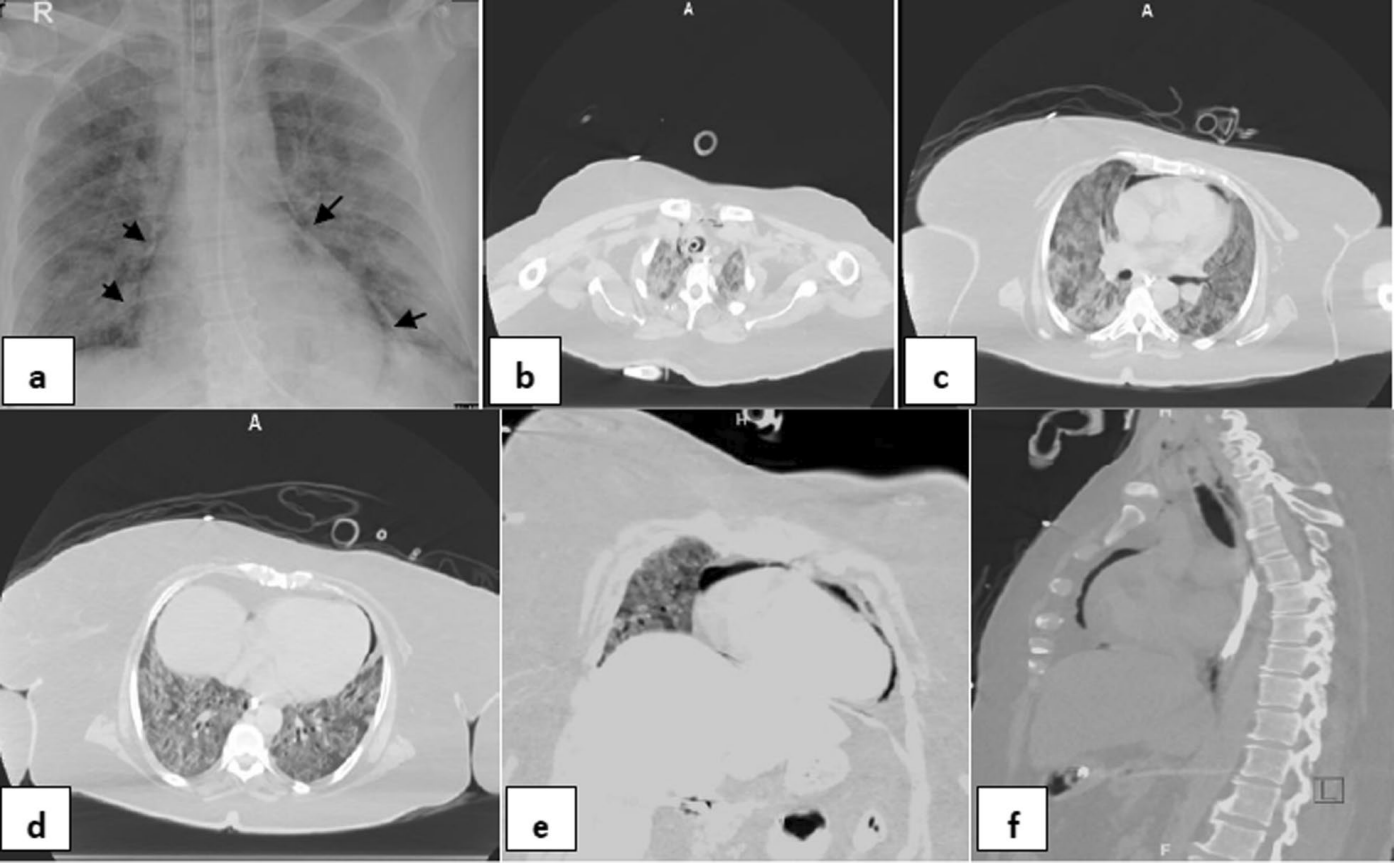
52-year-old female without significant medical history was intubated 7 days after admission, and developed pneumopericardium 4 days after intubation. a Frontal chest radiograph depicts mediastinal air bilaterally not exceed to the root of great vessels (black arrows) indicates air within pericardial sac. Note diffuse hazy interstitial lung markings and ill-defined air space opacities bilaterally. b–d series axial CT cuts of the chest, e and f coronal and sagittal reconstructions images, respectively, confirming the X-ray finding
